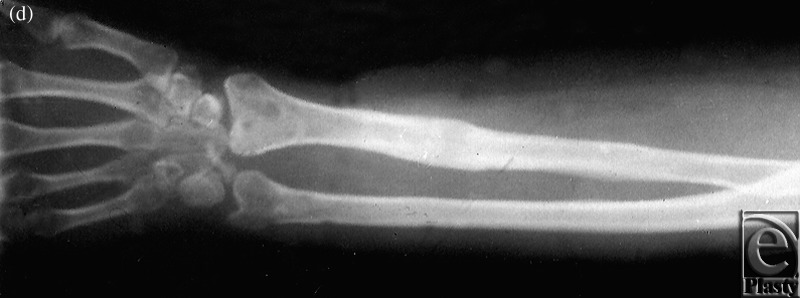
Case 4: Tumor. (*a*) Fibula osteocutaneous flap, (*b*) The 8-month postoperative view, (*c*) An 8-month postoperative x-ray, and (*d*) The 2-year postoperative view.
